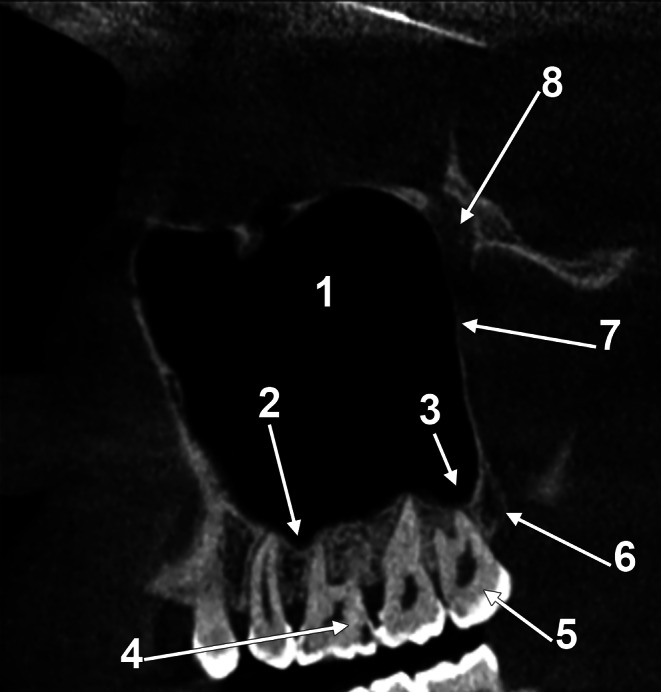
Orthosagittal CBCT slice through the alveolar process of the maxilla. A posterior sinus floor recess enters the alveolar tuberosity. (1) maxillary sinus; (2) mesial basin of the sinus floor; (3) distal basin of the sinus floor; (4) first upper molar; (5) third upper molar; (6) alveolar tuberosity; (7) maxillary tuberosity; (8) pterygopalatine fossa
